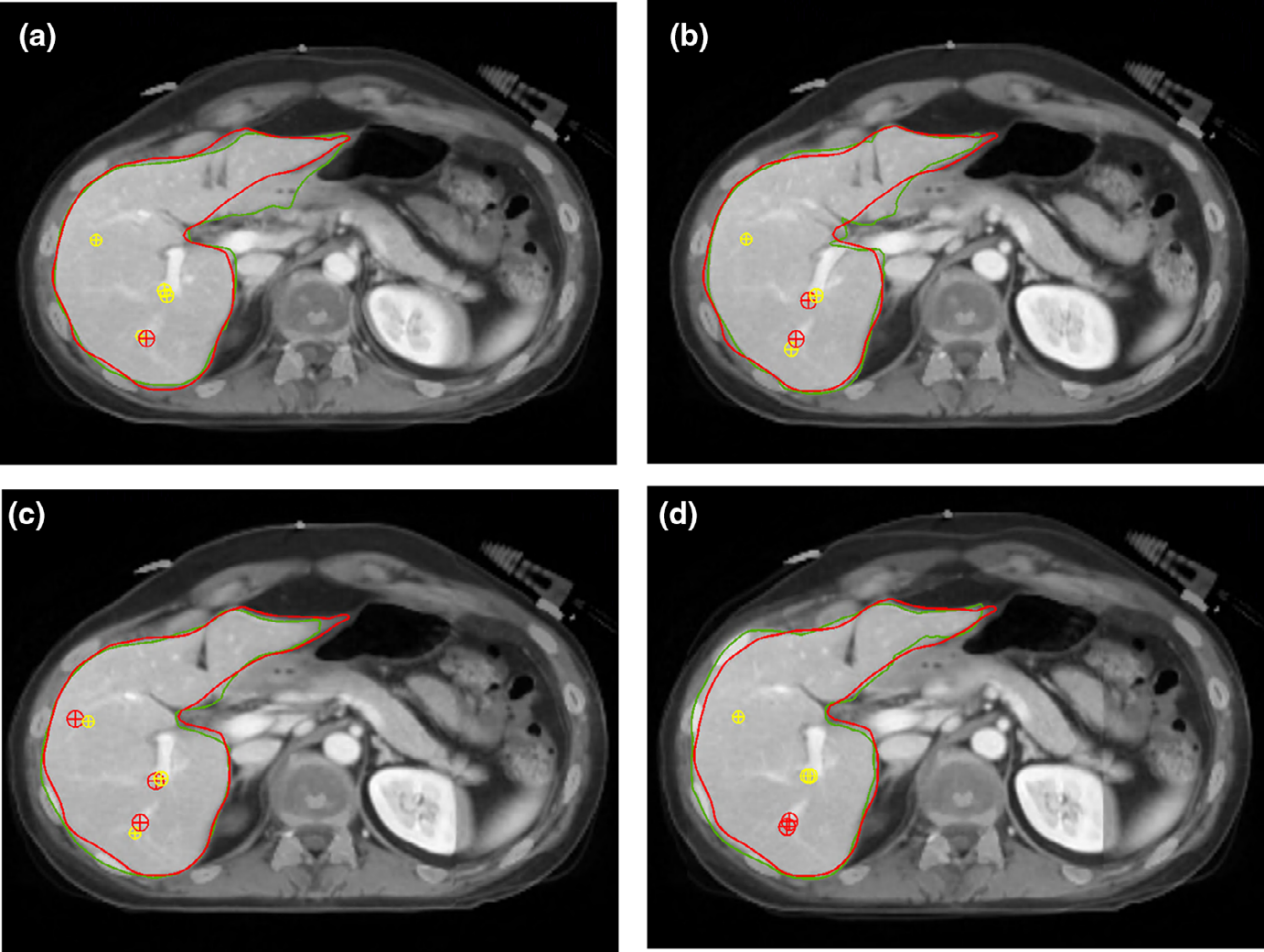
Planning CT and post‐SBRT MRI a) rigid registration b) DIR c)SG‐DIR_liver_, and d) SG‐DIR_liver+landmarks_. Corresponding landmarks are depicted by crosshairs, red contour corresponds to the liver structure on the planning CT, and green contour corresponds to the liver structure on the post‐SBRT MRI. SG‐DIR, structure‐guided deformable image registration.
